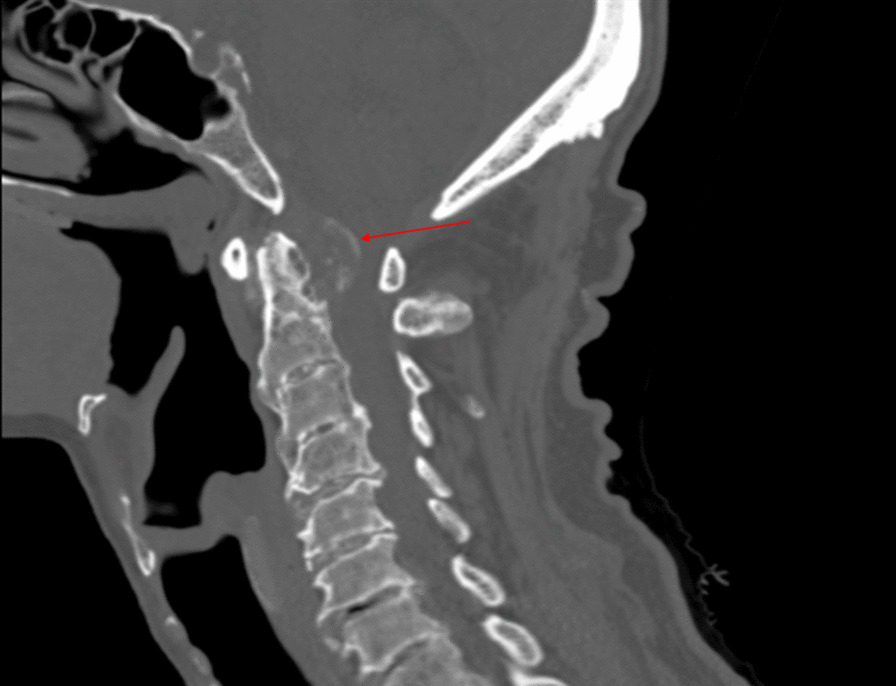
Sagittal computed tomography of cervical spine revealing a large, retrodental calcific mass following the contour of the transverse ligament of atlas, causing severe spinal canal stenosis. The radiographic finding is highlighted by the arrow
